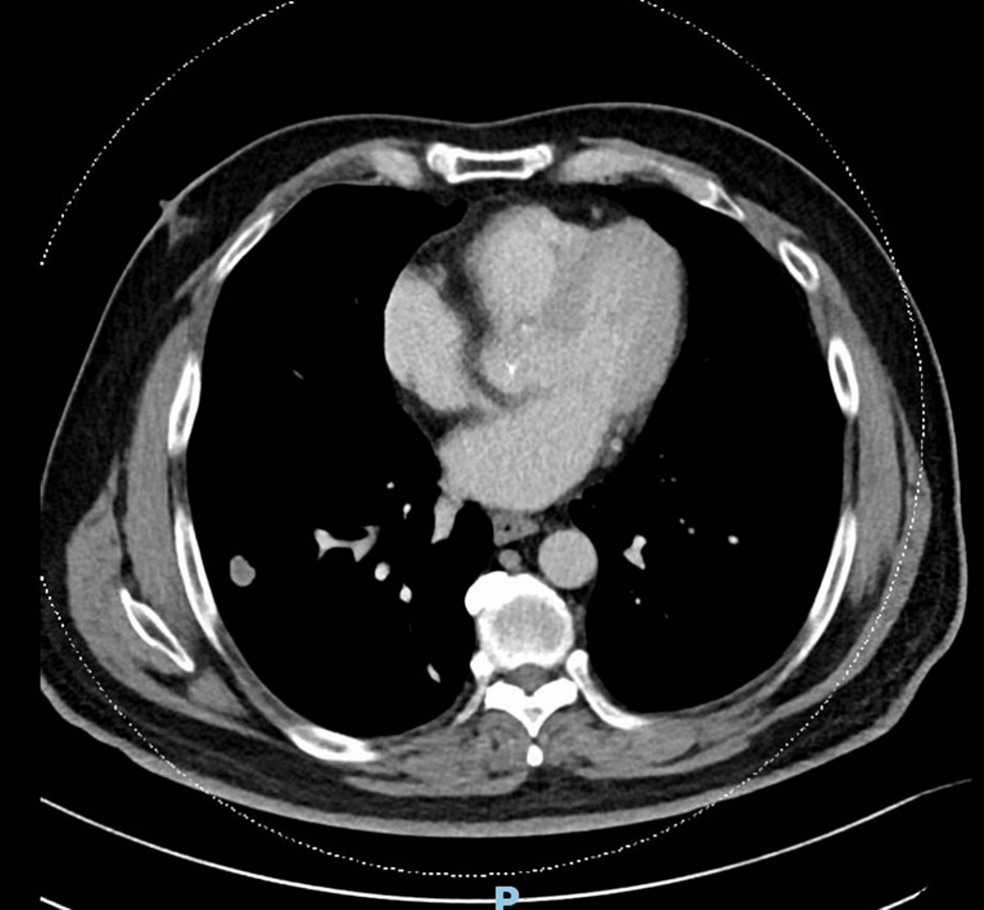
Computed tomography of the thorax showing resolution of the mass-like consolidation after corticosteroid treatment. The original lung nodule remains unchanged.
